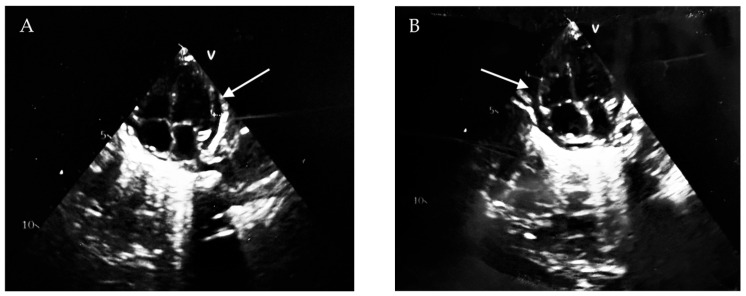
(A,B) Echocardiography of Patient 1 after pericardiocentesis—a residual pericardial effusion is visualized, mainly around the right ventricle (white arrows).
